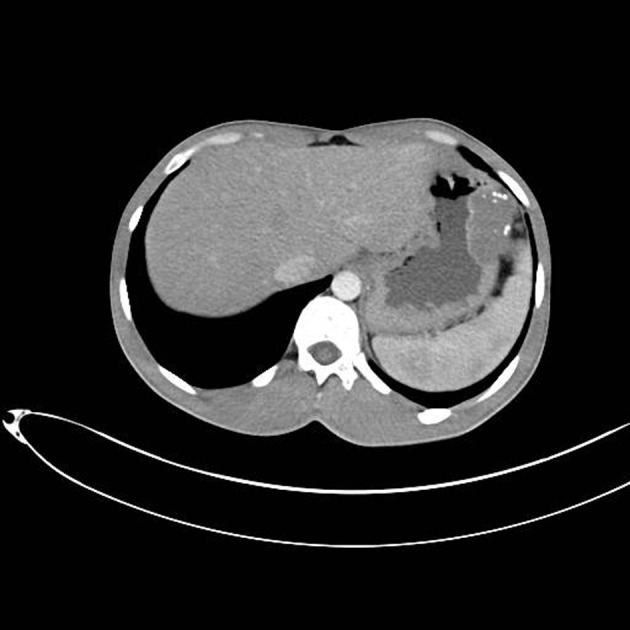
Computed Tomography image of gastric submucosal tumor. CT reveals an isodense and smooth contoured mass lesion that contained focal calcifications and was located on the greater gastric curvature in the left upper abdominal quadrant.
